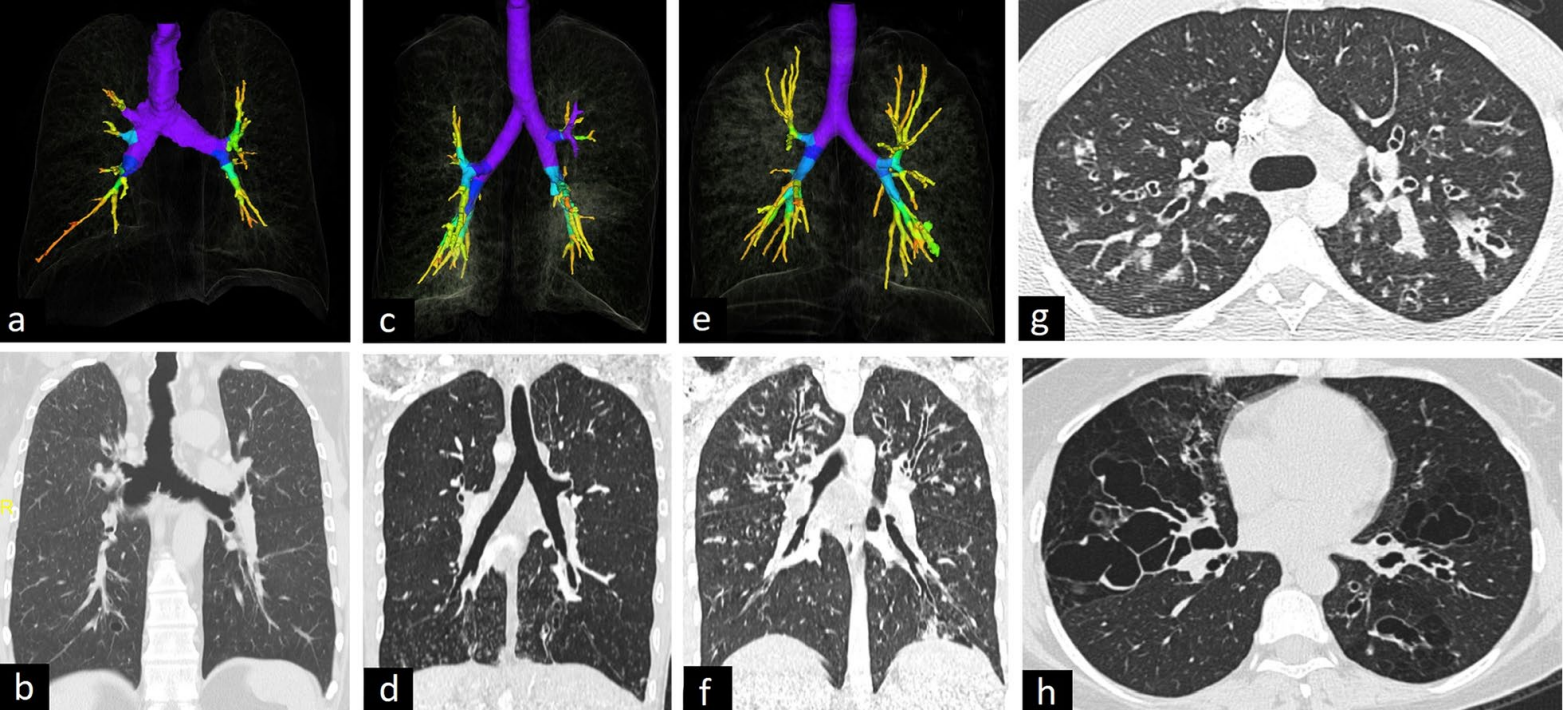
Imaging findings of congenital diseases causing bronchial dilatation. 3D reconstruction (a) and coronal CT image (b) show dilatation of the main airways in a patient with Mounier-Kuhn syndrome. Bronchiectasis, centrilobular opacities, and increased density predominantly affecting the lower lobes of the lungs are seen in a patient diagnosed with Kartagener (c, d). Upper lobe-predominant bronchiectasis and thickened bronchial walls are observed in cystic fibrosis (e–g). Severe and bilateral cystic bronchiectasis in the subsegmental bronchi (4th and 6th generation) is seen in a patient with Williams–Campbell syndrome (h)
